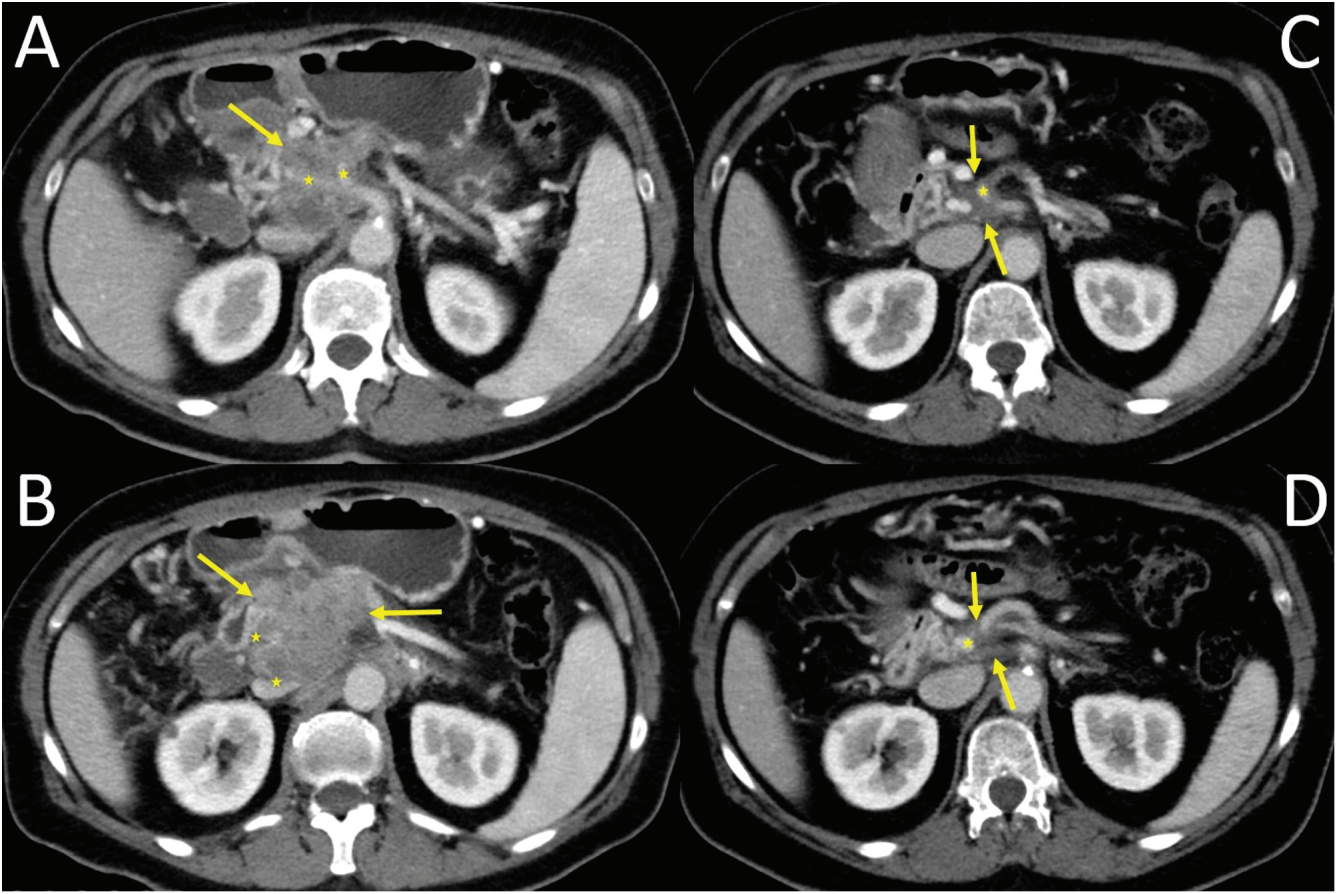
A case of a patient with PDAC with confirmed *BRCA2* mutation. CT in porto-venous phase, axial planes. Images A and B show a large pancreatic tumour (arrows) before treatment (classified as cT4N1M1), which infiltrates the branches of the ceoliac trunk (asterixis), inferior vena cava (asterixis) and completely thromboses the portal vein (asterixis). After treatment (C, D), the original pancreatic infiltrate was significantly reduced in size (arrows; classified as ycT4N0M0, but CT cannot reliably distinguish residual tumour changes from post-treatment changes), pathologic densities continue to contact the branches of the coeliac trunk (asterixis) and stenotize the portal vein (asterixis).
